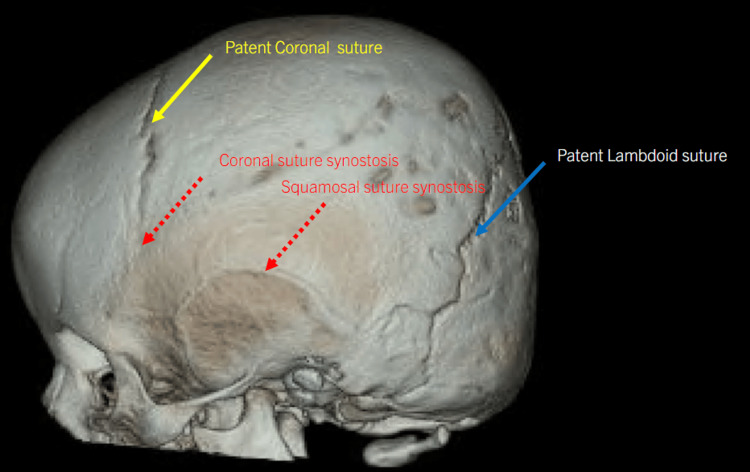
CT scan and three-dimensional reconstruction of the skull demonstrating microcephaly and craniosynostosis of the metopic, squamosal, and bicoronal (partial) sutures and patent lambdoid suture.
